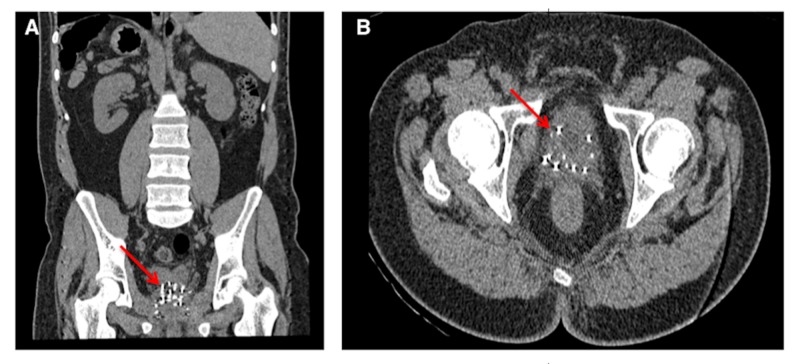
Coronal (A) and axial (B) CT of the abdomen and pelvis without contrast showing multiple 4 mm x 0.8 mm densities within the prostate gland, consistent with brachytherapy seeds.
